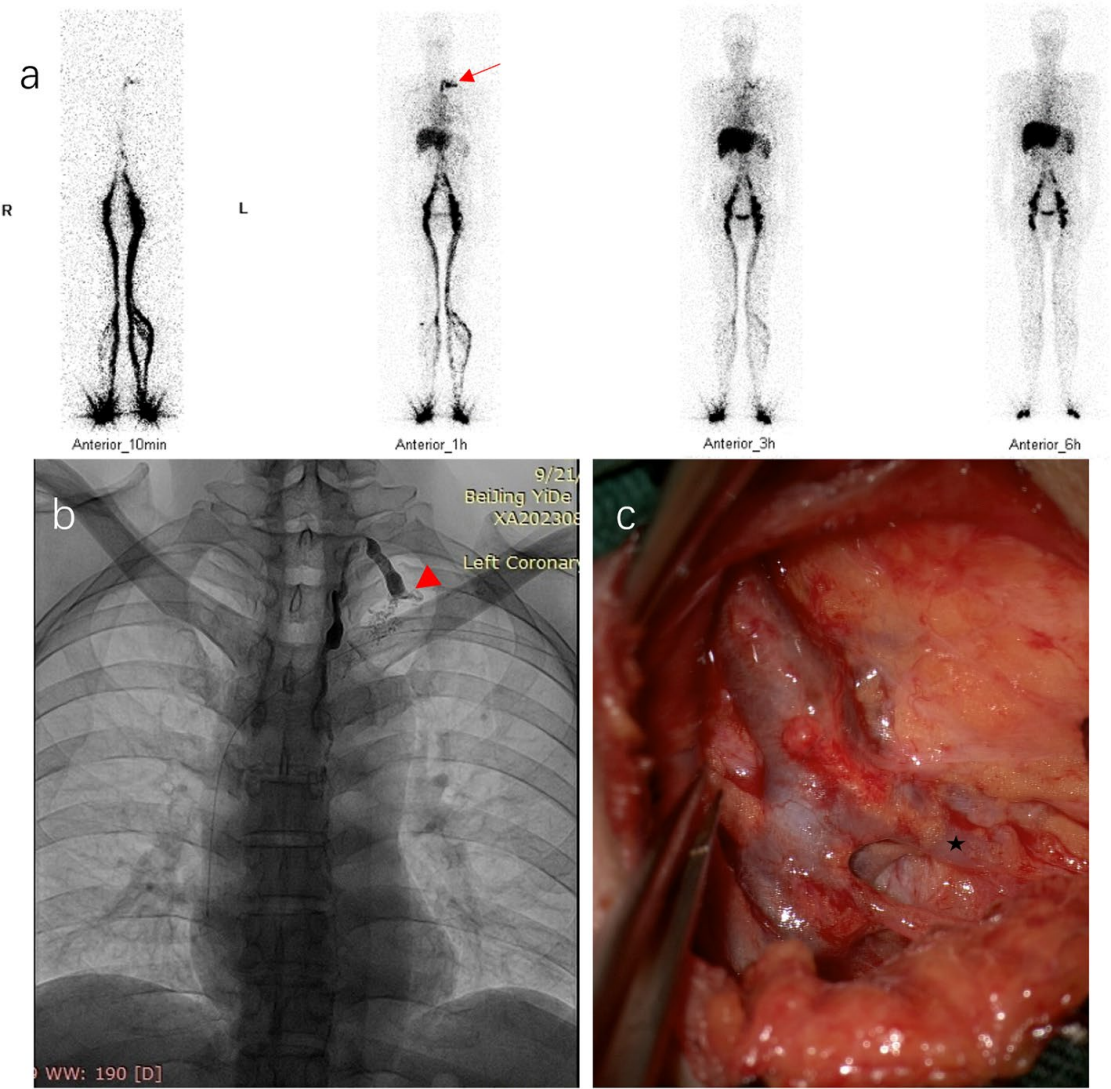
Functional obstruction of thoracic duct outlet. The patient suffered hepatic lymphorrhea after laparoscopic radical gastrectomy. Lymphoscintigraphy (a) showed temporary left subclavian-jugular venous angle radioactivity (arrow). Lymphangiography (b) demonstrated a distended cervical thoracic duct and ampulla (arrowhead), raised pressure in the duct, and increased flow rate into the vein. Lymph flow through thoracic outlets was mildly restricted and reflux was slight (arrowhead). The thoracic duct and subclavian lymphatic trunk (star) were distended (c)
